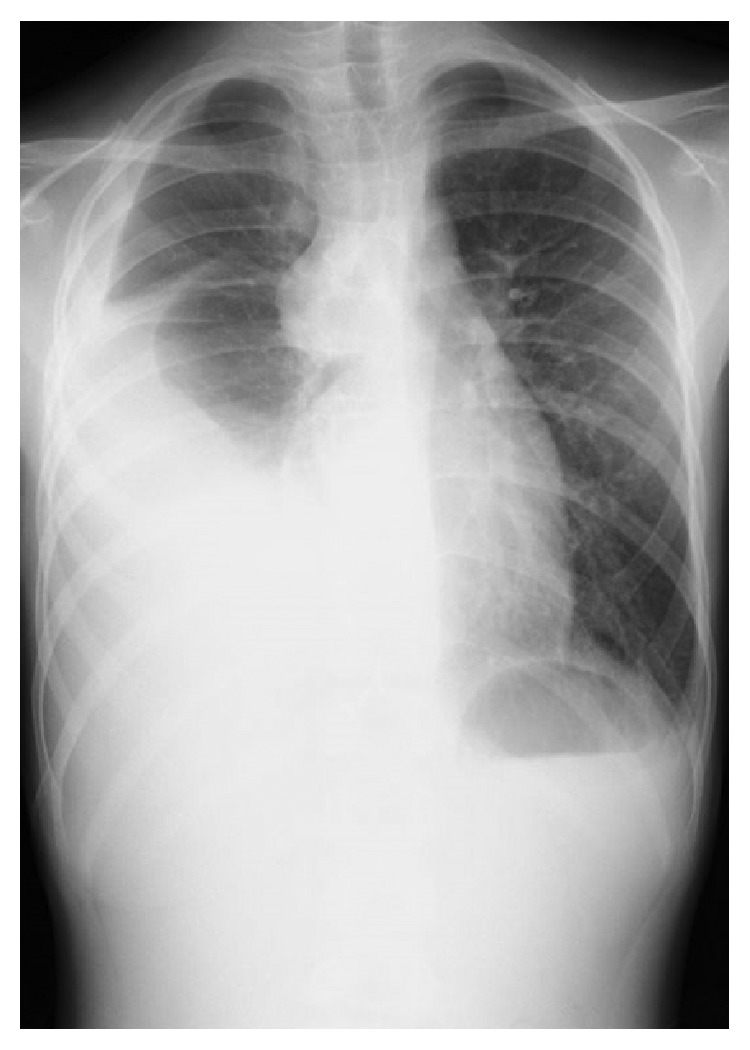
A radiograph showing massive pleural effusion in the right lung.
